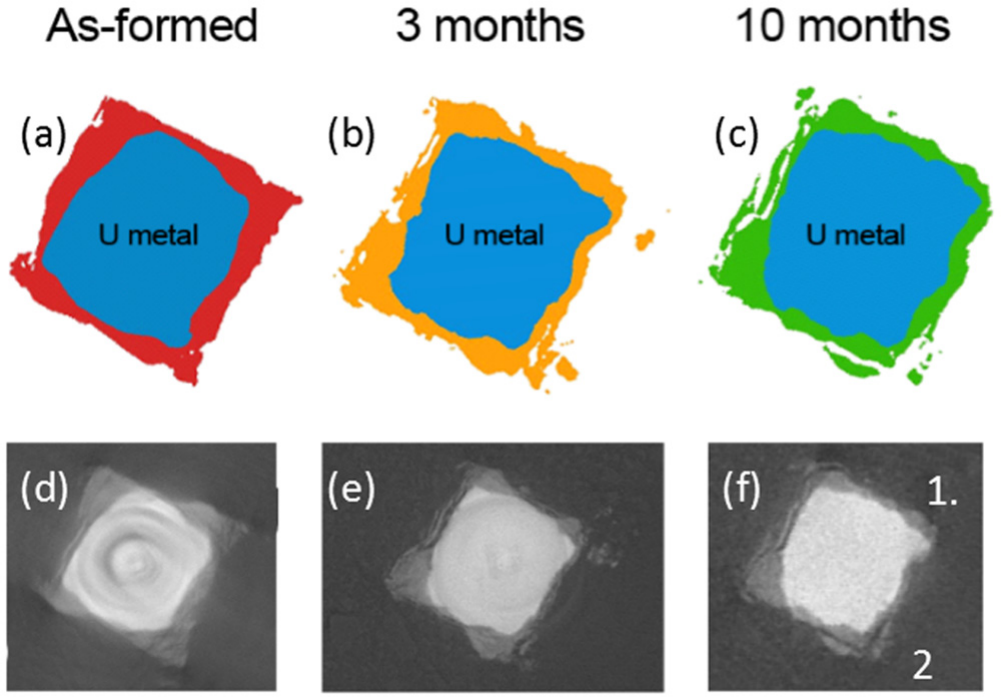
Cross sections at the same position of the uranium encapsulated in grout over time. Images (a) to (c) are accurate representations of the uranium (blue) and surrounding corrosion products (red, orange and green) perpendicular to the length of the sample. Images (d) to (f) are radiographs corresponding to the above representations, but with the additional surrounding grout.
